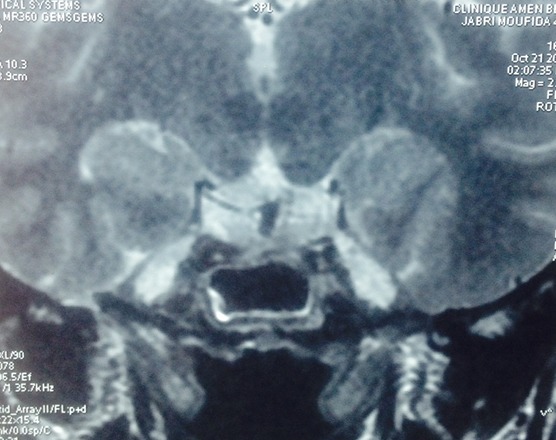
Magnetic resonance imaging (MRI) demonstrated a macroadenoma with the size of 1.35 ×2.9 ×1.2 cm, without a suprasellar extension or a compression of the optic chiasma. It encased the left cavernous sinus and internal carotid artery
